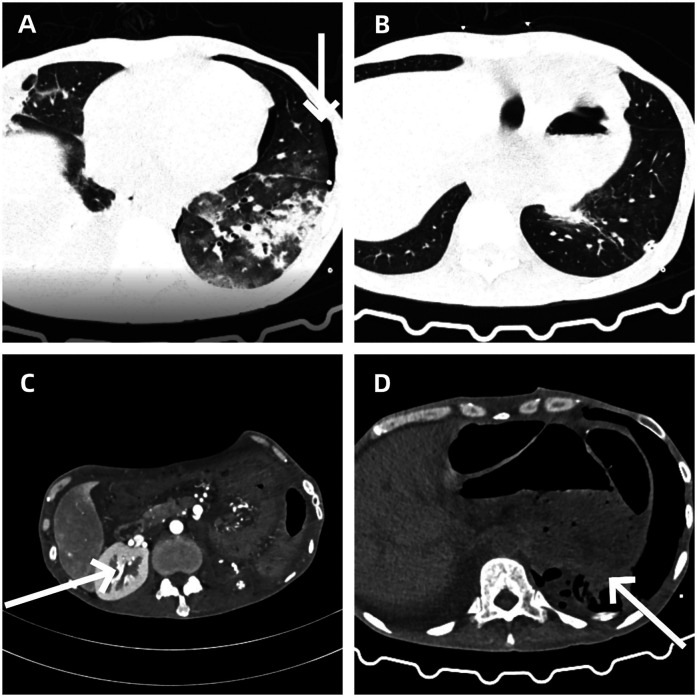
(A) Left-sided pneumothorax as a postoperative complication. (B) Gradual resolution of pneumothorax following closed thoracic drainage. (C) Abdominal contrast-enhanced CT confirms complete resection of the tumor. (D) The resolution of the subphrenic space-occupying lesion.
